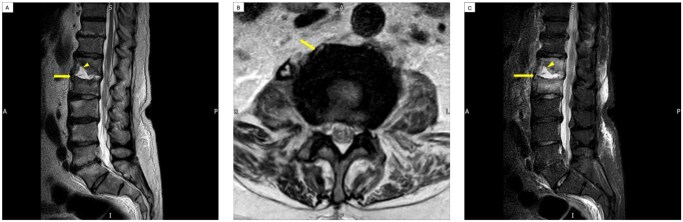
Sagittal T2 and axial T2 weighted images (A, B) and sagittal STIR sequences (C) from subsequent MRI lumbar spine showing T12/L1 disc liquefaction with partial collapse of the inferior endplate of T12/L1, small anterior erosions and marked bone marrow oedema.
